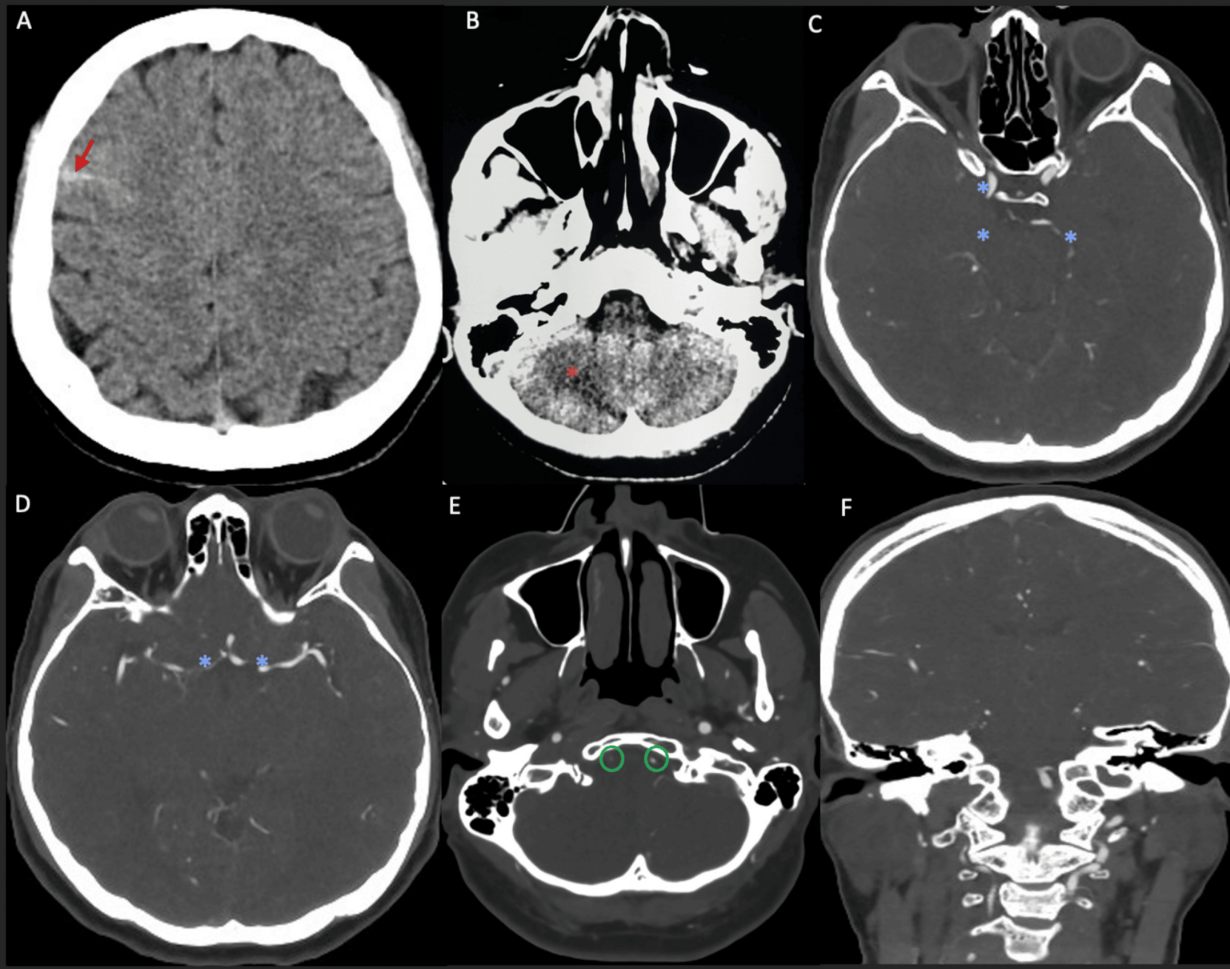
Non-contrast CT of the brain and CT angiography at admission (A–F) (A, B) Non-contrast CT of the brain demonstrates a small subarachnoid hemorrhage in the right high frontal region (red arrow) and an ill-defined hypodensity within the right cerebellar hemisphere (orange asterisk), concerning for acute infarction. (C–F) CT angiography shows multifocal segmental narrowing of the right internal carotid artery, predominantly involving the cavernous and supraclinoid segments, with additional multifocal narrowing of several vessels of the circle of Willis, including the bilateral anterior cerebral (A1), middle cerebral (M1), and posterior cerebral arteries (blue asterisks). Focal areas of narrowing are also noted in the distal segments of both vertebral arteries, with complete occlusion of the distal right vertebral artery and non-opacification of the right posterior inferior cerebellar artery (PICA) (green circles).
